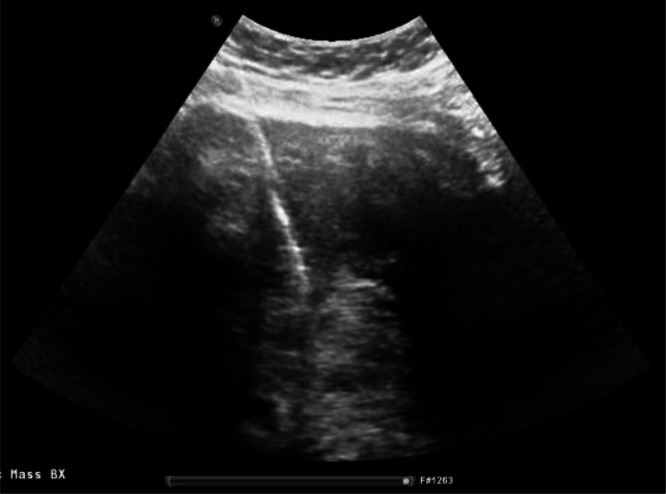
Ultrasound-guided core needle biopsy confirmed the position of the needle within the mass, which was diagnosed as AAM.
